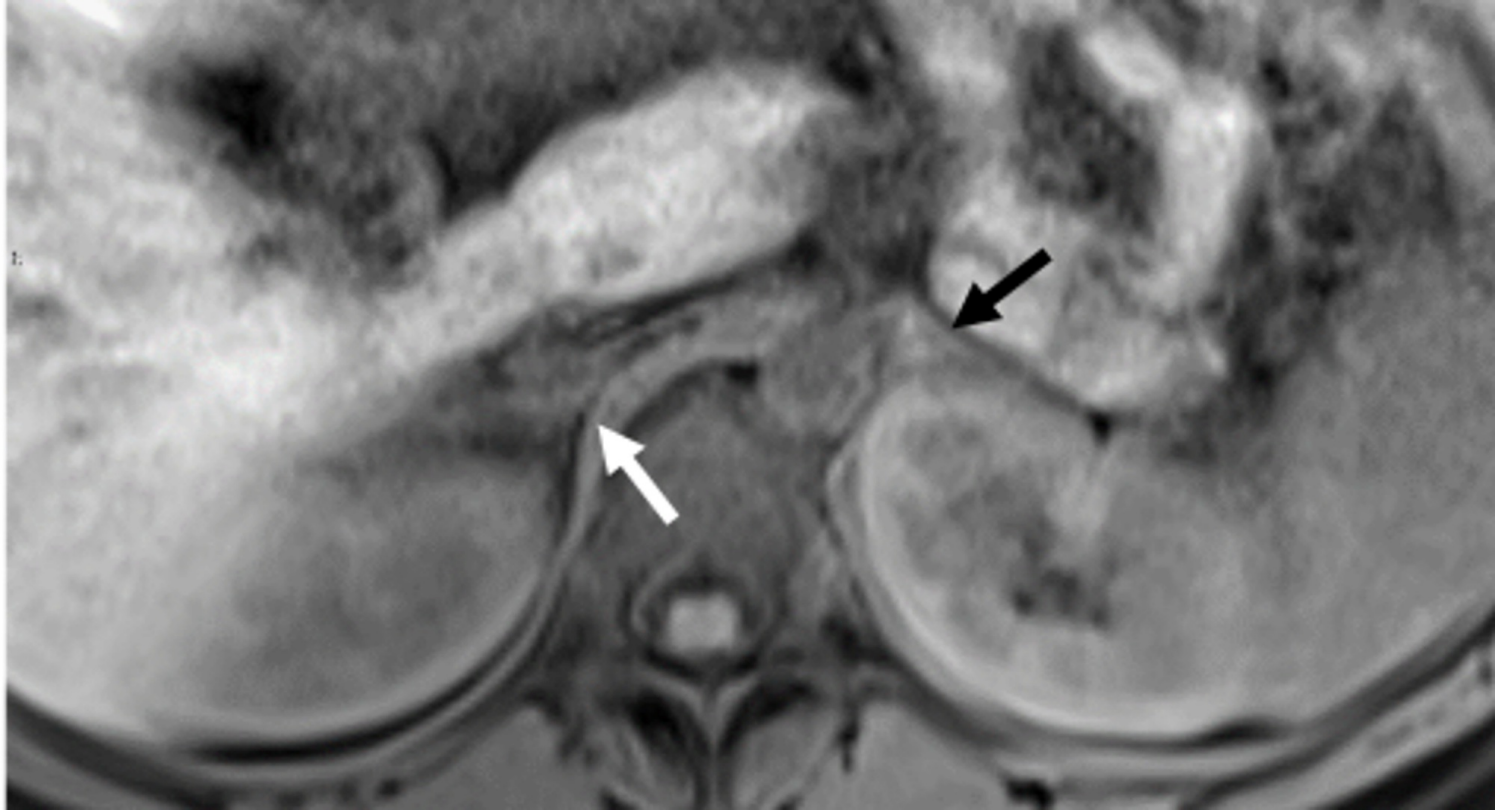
T1-weighted Image at the level of the adrenal glands. T1-weighted fat saturation image showing a diffusely swollen right adrenal gland of intermediate to hypointense signal (white arrow). The left adrenal gland is isointense without edema (black arrow).
